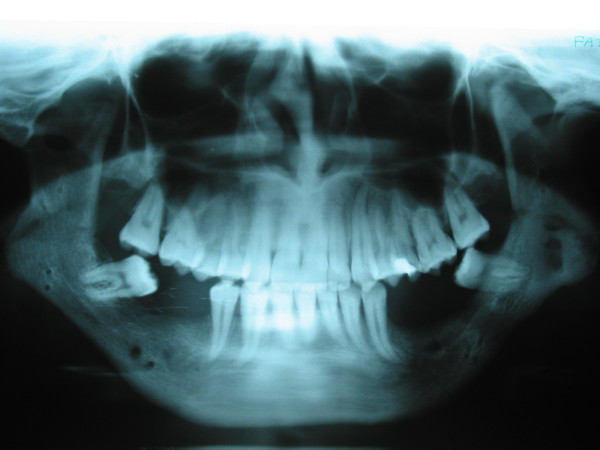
Radiograph taken after consolidation phase showing the formation of new bone at the distraction site.
